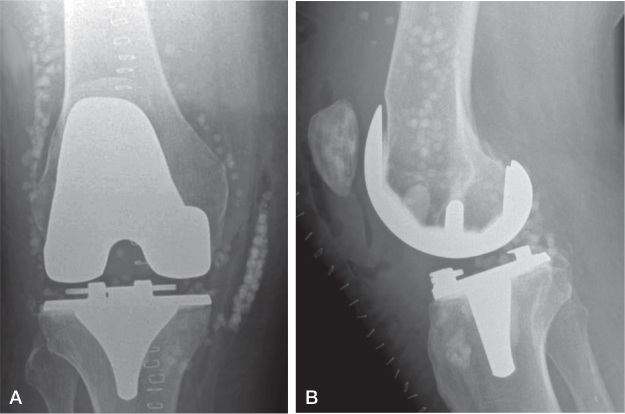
(A) Anteroposterior and (B) lateral radiograph after a DAIR procedure with application of calcium sulphate beads of 10 mL antibiotic-loaded rapid cure powder mixed with 1 g vancomycin and 240 mg gentamicin for an acute hematogenous periprosthetic knee infection with *Streptococcus dysgalactiae* in a 68-year-old male patient.
